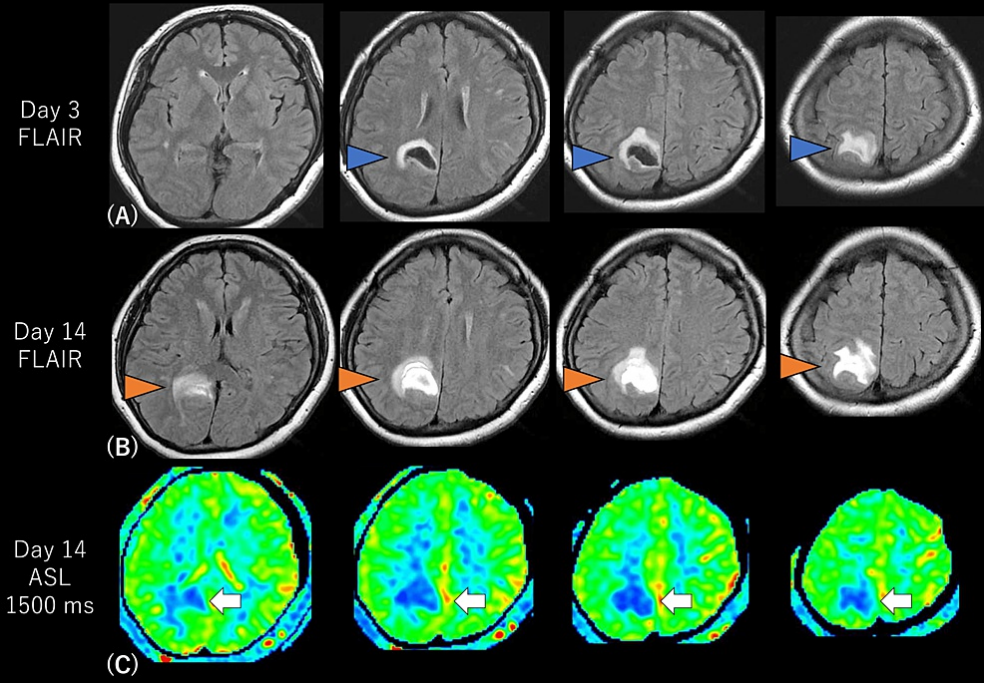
Radiological findings. The right parietal subcortical hematoma and surrounding edema showed on day 3 FLAIR images (blue arrowheads in A). The edematous lesion and hematoma remained on day 14 FLAIR images (orange arrowheads in B). The ASL images revealed hypoperfusion in the right parietal lobe (arrows in C). FLAIR: fluid-attenuated inversion recovery; ASL: arterial spin labeling.
